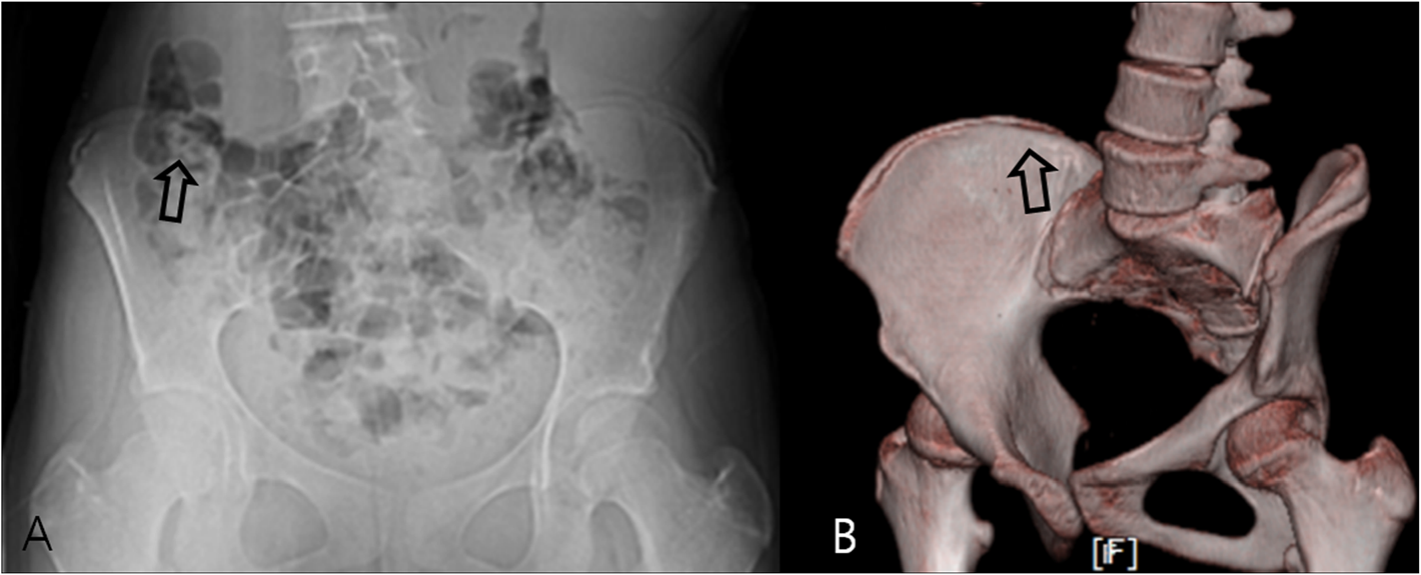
Gas shadow. (A) Plain radiography performed on a 13-year-old girl, a gas shadow on the posterior iliac crest prevented interpretation of bilateral excursion of the apophysis, and the patient was graded as Original Risser stage 2 and French Risser stage 1. (B) A 3D-CT image of the same patient, excursion of the iliac apophysis was clearly visible along the entire iliac bone, and was graded as Original Risser stage 4 and French Risser stage 3. Gas and internal organ shadows interfered with accurate staging.
